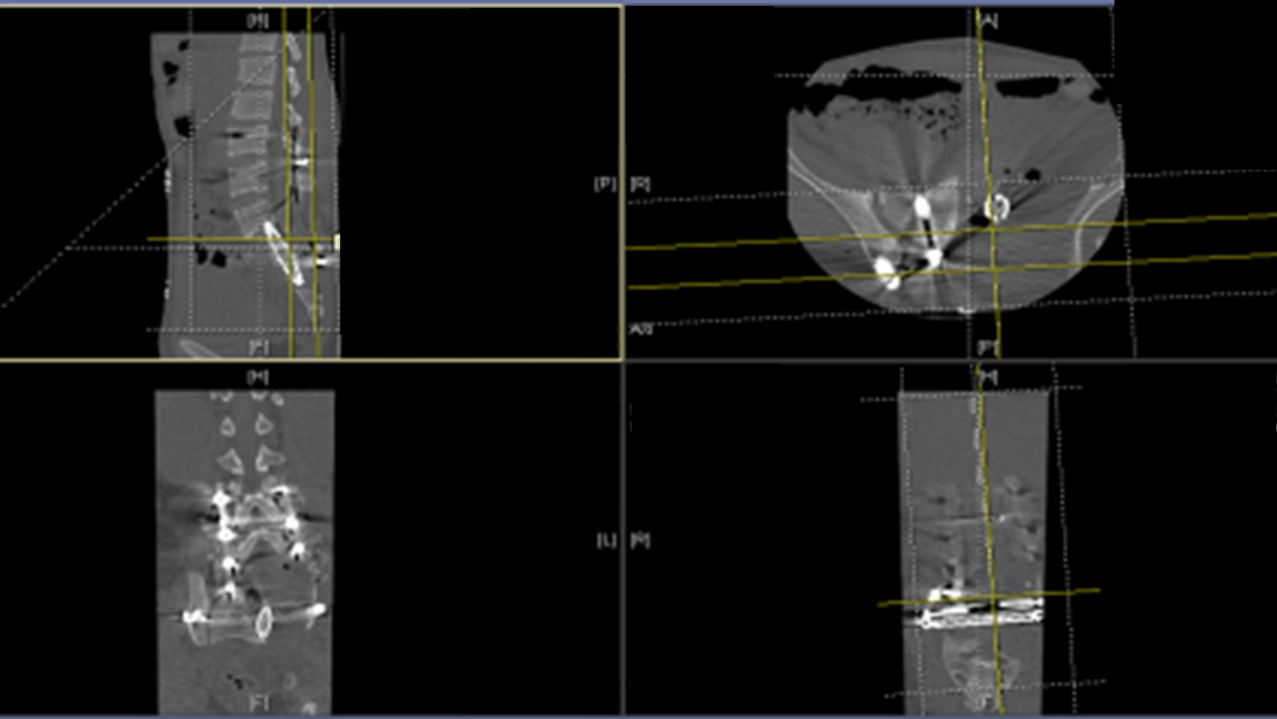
A postoperative CT showing the complex tumor resection with sufficient resection margins.
